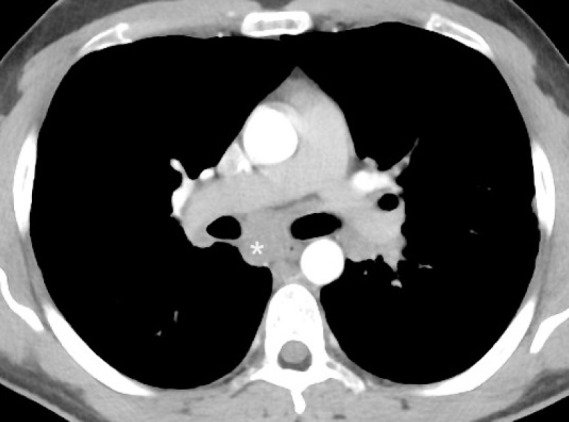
Post contrast CT of chest of a patient with history of lymphoma shows a soft tissue density mass (asterisk) in the sub-carinal region suggesting lymphadenopathy. Note that the difference in the enhancement of the aorta and pulmonary trunk and its branches is due to timing of acquisition of image after the administration of radiographic contrast
